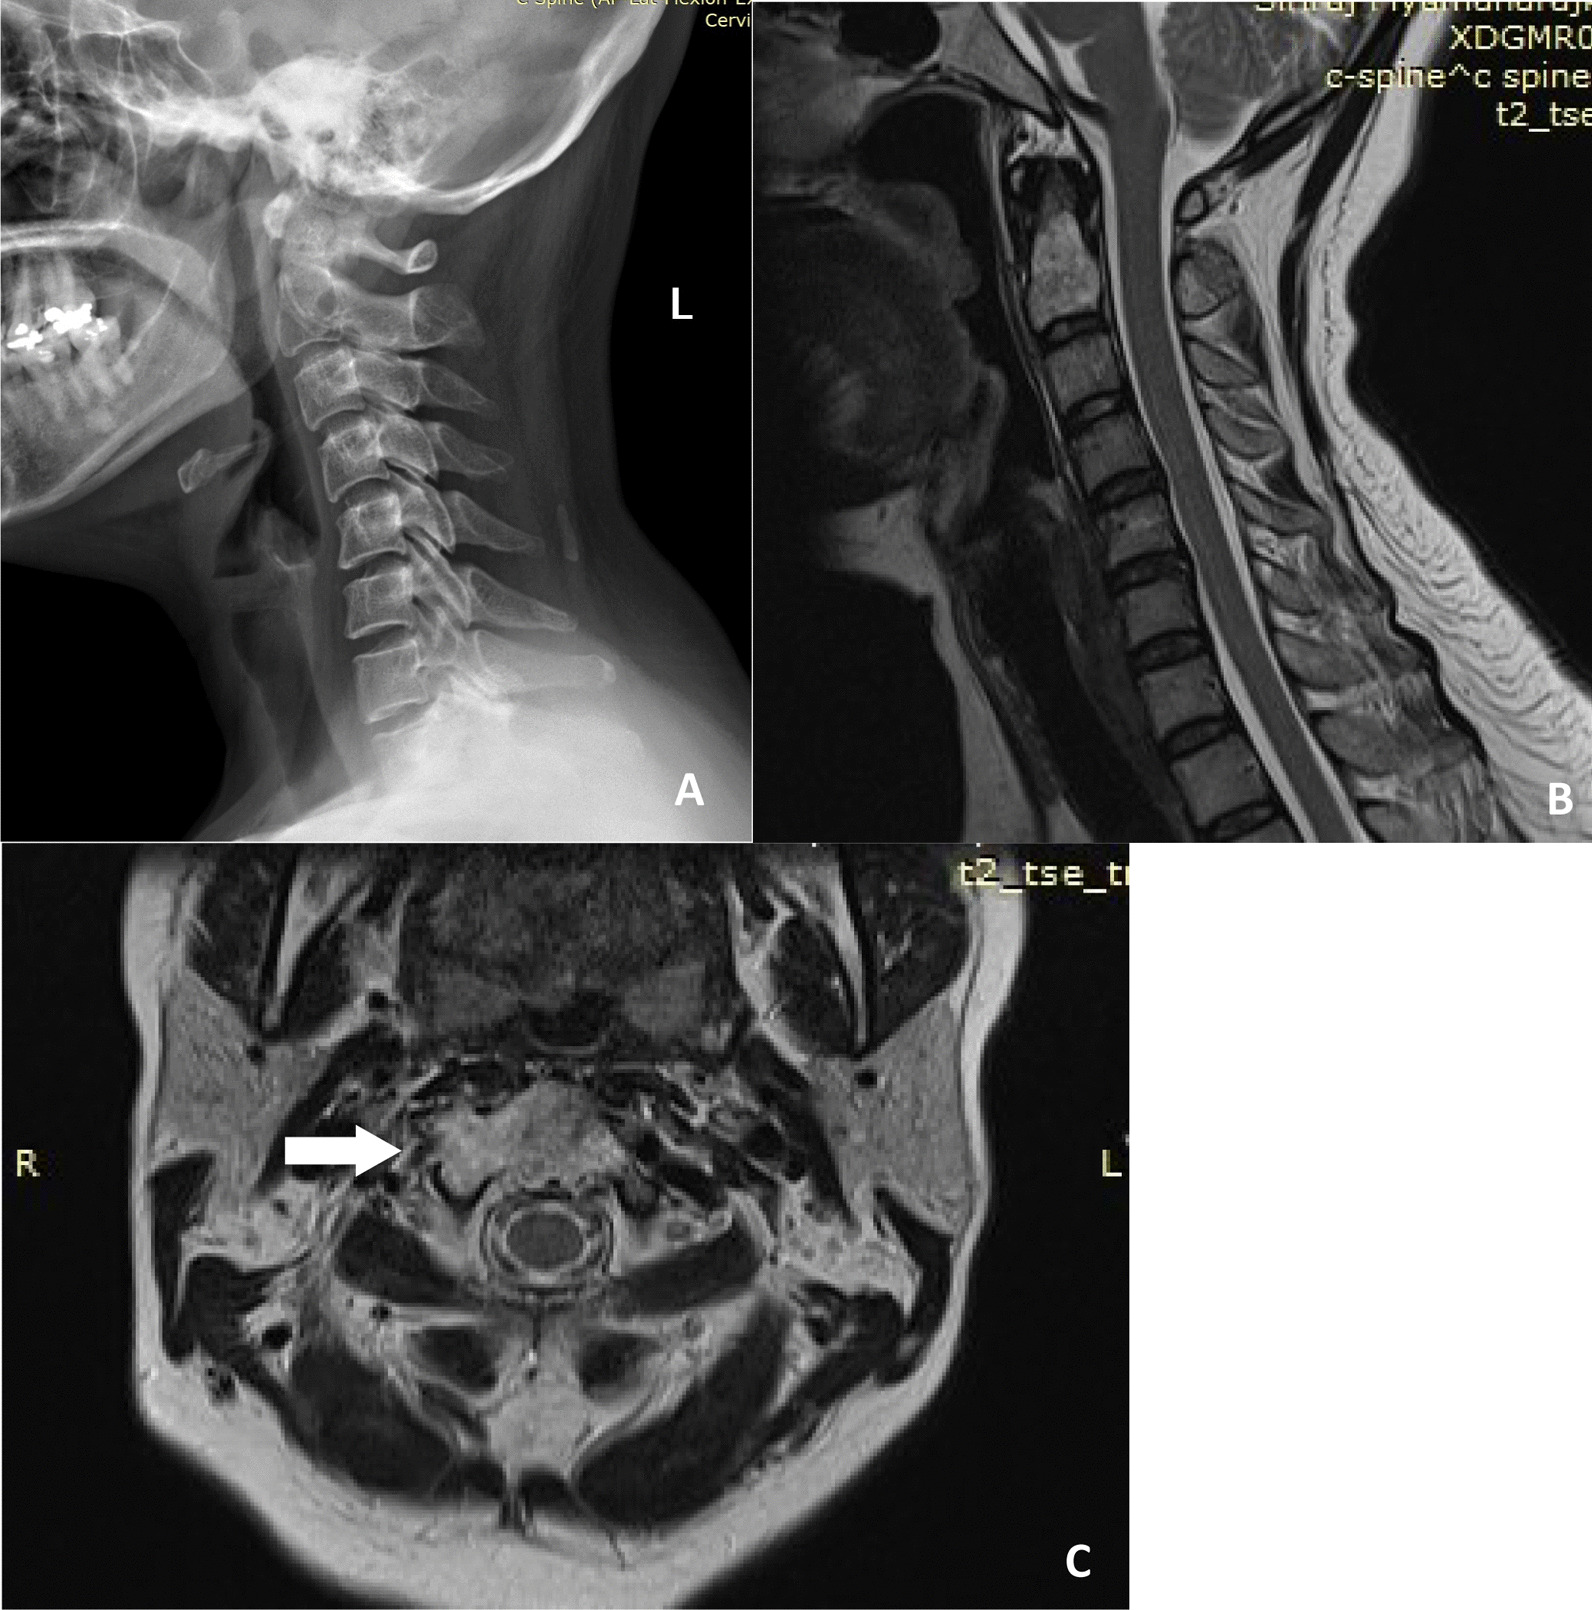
Preoperative plain cervical spine radiograph showing loss of cervical lordosis and mild narrowing at the C5–6 intervertebral discs (A) and T2-weighted magnetic resonance images (sagittal section, B; axial section, C). The magnetic resonance imaging revealed a heterogeneous mass involving C2, with an extension to the right foramen transversarium (white arrow)
